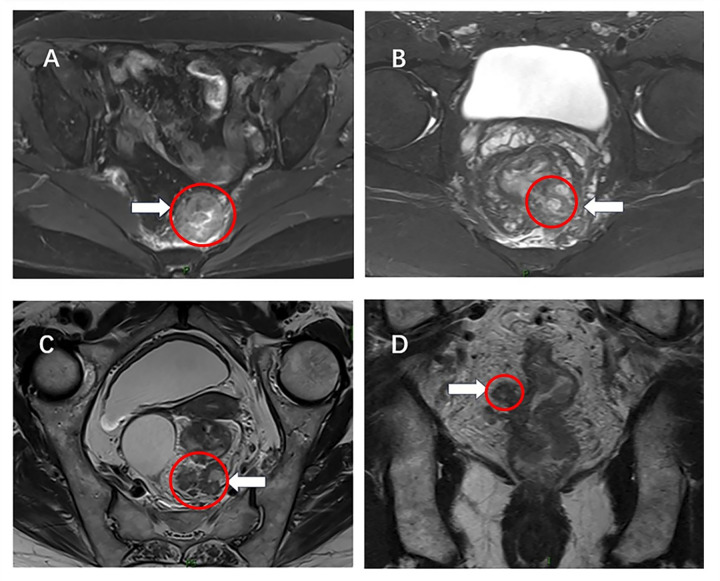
Axial T2-weighted image. (A) demonstrating tumor deposit (white arrow) with irregular shape, signal heterogeneity and is seen invading the anterior sacral fascia. (rectal cancer has not been shown in the image). (B) demonstrating tumor deposit (white arrow) with irregular shape, signal heterogeneity and irregular margins and is seen invading the mesorsstal fascia. (C) from another patient depicting multiple tumor deposits (white arrow) which are close to the mesorsstal fascia. Coronal T2-weighted image (D) from another patient depicting a tumor deposit (white arrow) with subcircular shape and smooth margins.
